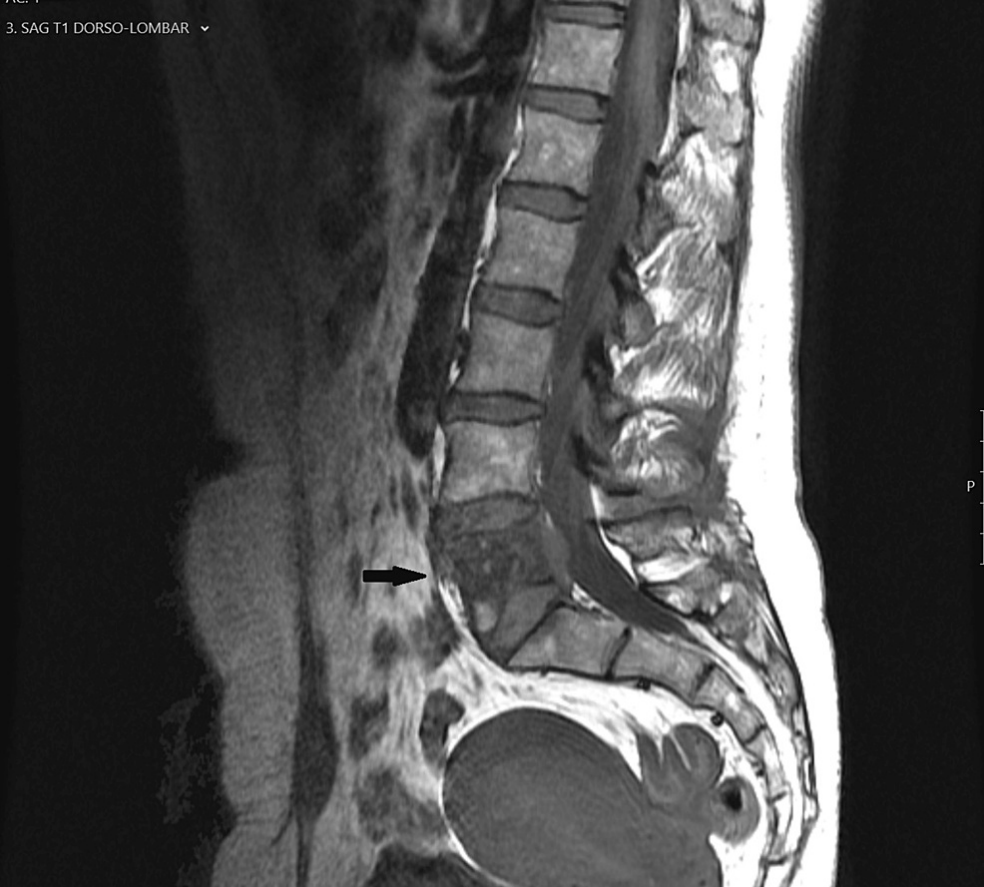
MRI, T1WI sequence. On MRI, the lesion was isointense on T1WI.
